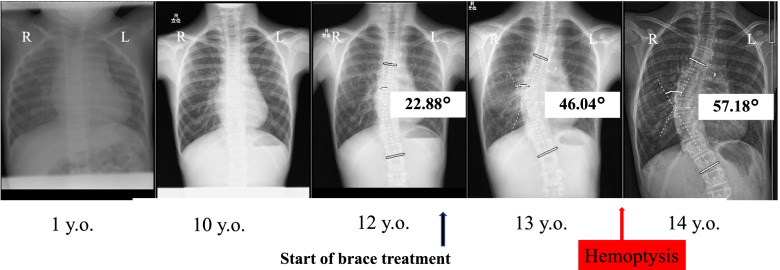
Changes in preoperative radiographs. At age 13, her main thoracic curve had a Cobb angle of approximately 46 degrees, with episodes of hemoptysis
